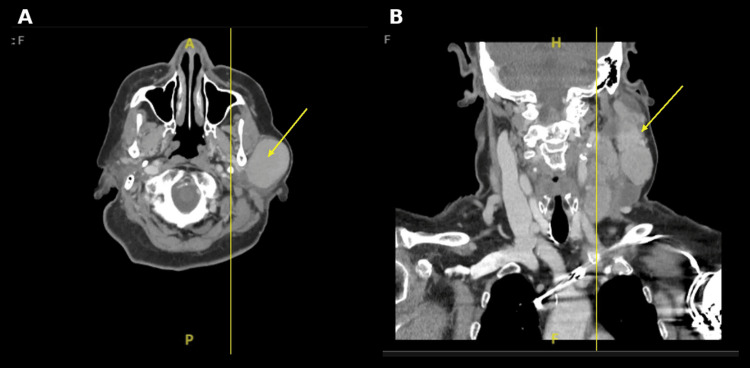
Contrast-enhanced computed tomography of the neck, demonstrating left cervical lymphadenopathy. (A) Axial view showing an enlarged, heterogeneous left cervical lymph node (arrow). (B) Coronal reconstruction demonstrating bulky left cervical adenopathy (arrow), most prominent at levels II and III. Multiple enlarged lymph nodes measured up to 4.3 cm with features concerning necrosis.
